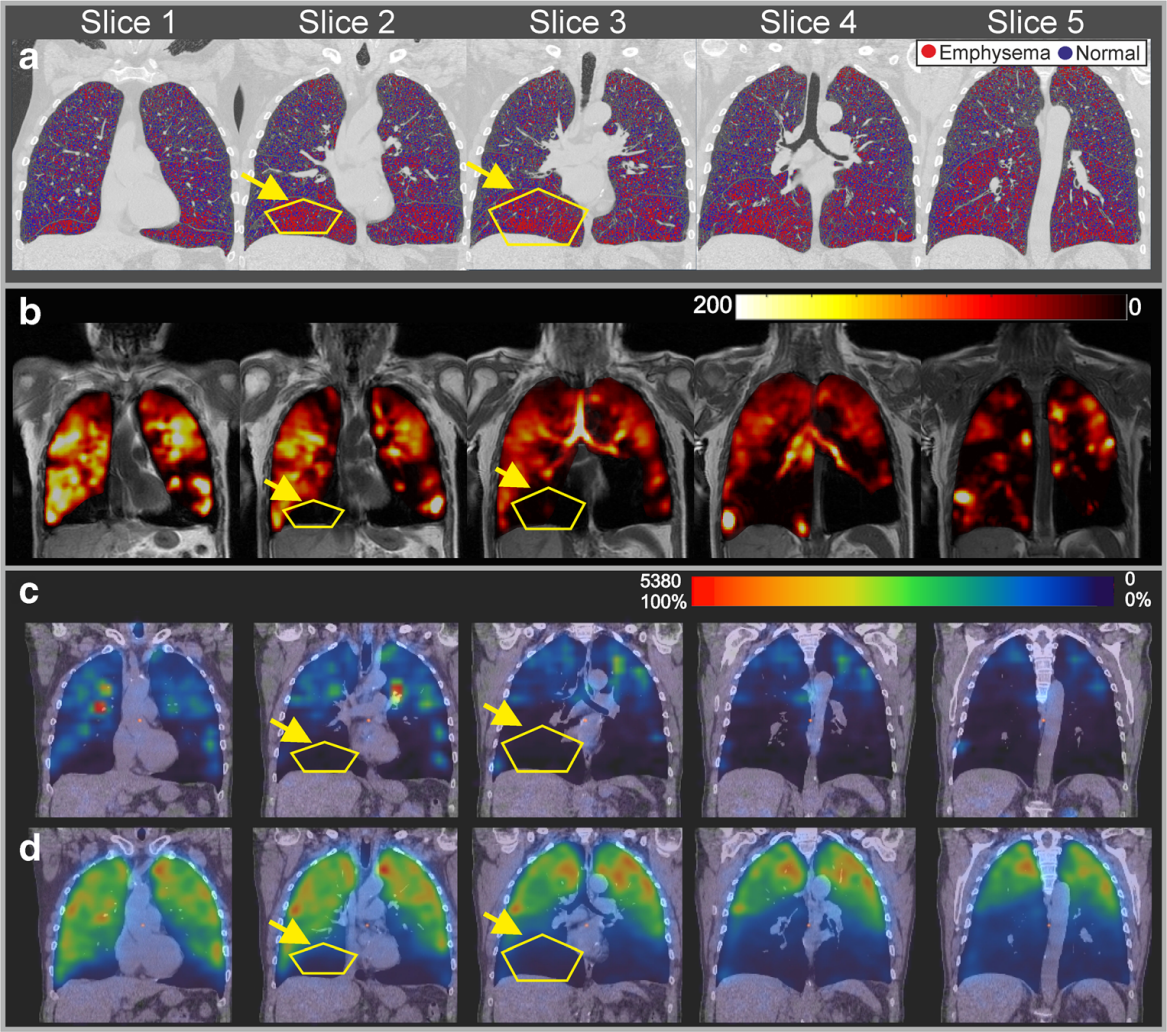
a The high-resolution CT images of a stage IV COPD (subject 3 in Table 1) patient for selected five slices in coronal plane including the measured emphysema values are shown. Yellow lines indicate the regions with high emphysema. b Proton MRI and HPX-MRI images of a stage IV COPD patient (subject 3 in Table 1) for selected five slices in coronal plane are shown. c, d V/Q-SPECT and low-dose CT fused images of a stage IV COPD patient (subject 3 in Table 1) for selected five slices in coronal plane are shown. Yellow lines indicate the regions with severe emphysema
